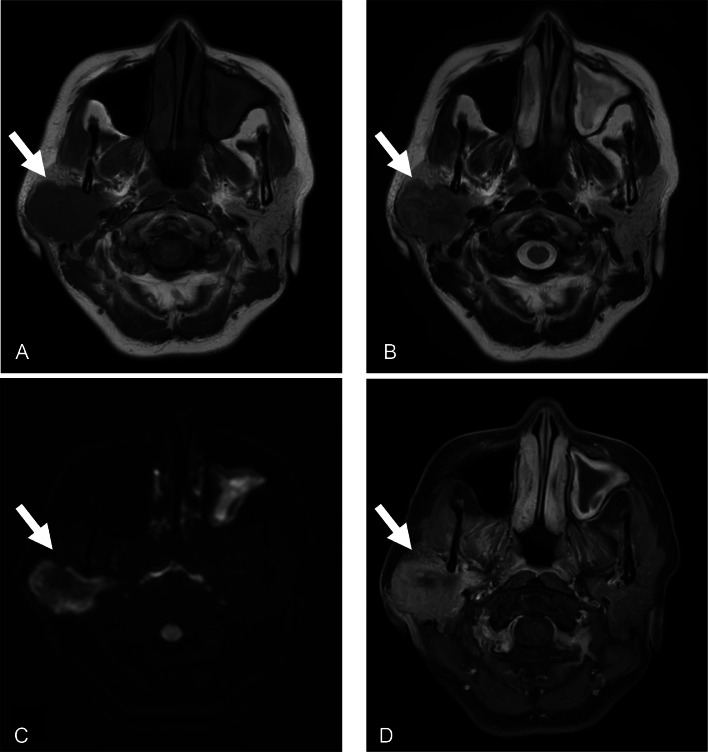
(A) Axial T1-weighted imaging revealed low signal intensity. (B) Axial T2-weighted imaging revealed heterogeneous low signal intensity. (C) Axial diffusion-weighted imaging revealed high signal intensity. (D) Contrast-enhanced T1-weighted imaging revealed a contrast effect with limbal predominance and a contrast-deficient area suspicious for internal necrosis.
